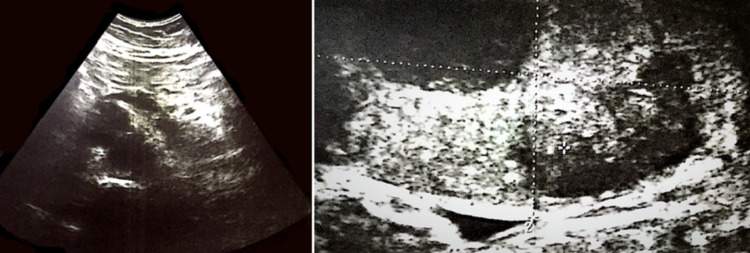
Testicular ultrasound showed two hypoechoic nodules in the right testis.
